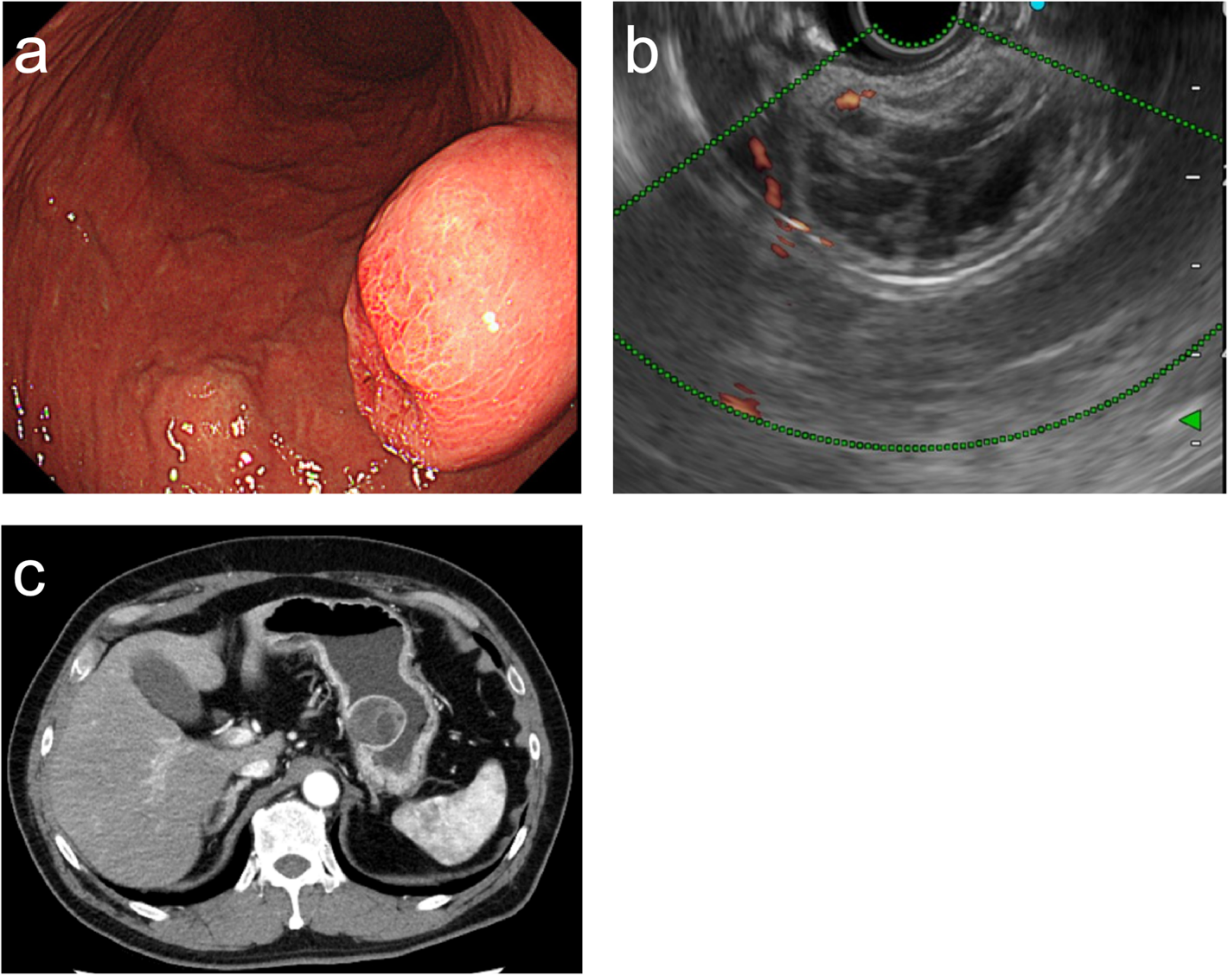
a Upper gastrointestinal endoscopy showed a gastric SMT with a central dimple at the posterior wall of the upper gastric body. b Endoscopic ultrasonography (20 MHz) showed heterogenous tumor measuring 35 mm in diameter with cystic spots. c An enhanced computed tomography scan revealed an intraluminal growth type tumor at the posterior wall of the upper gastric body
